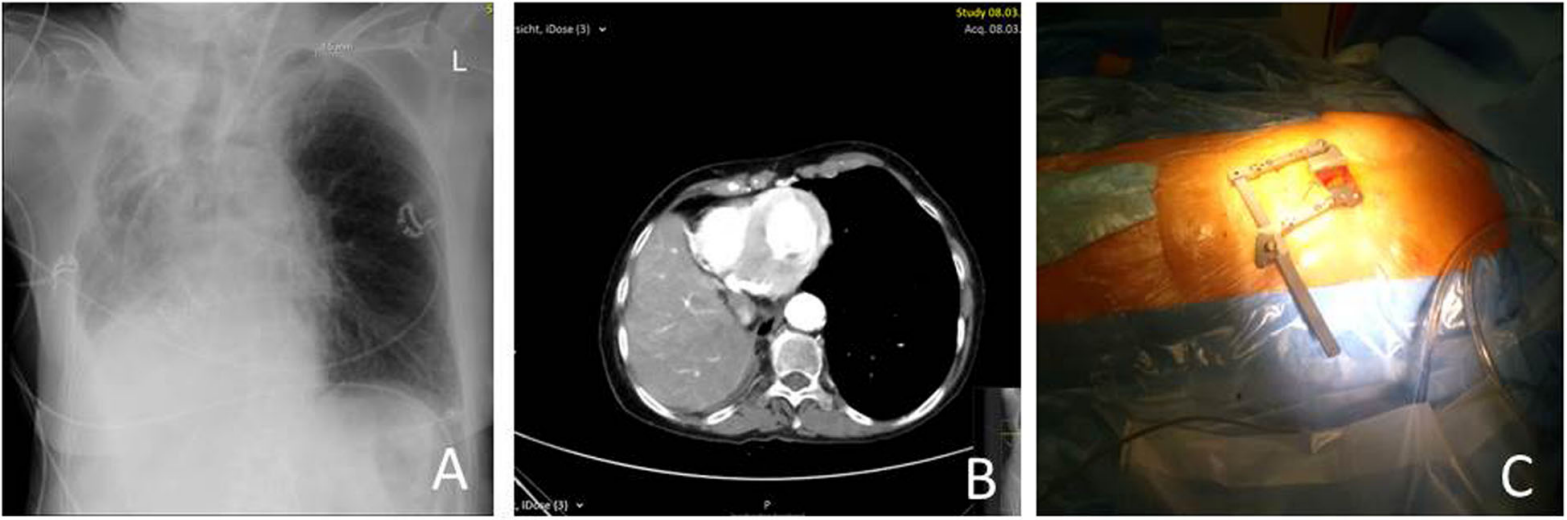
a and b showing rightward shift of the heart. The apex is located below the sternum. c shows the subxiphoidal incision through which TA-TAVR was performed. Arrow on the patient’s chest marks location of the apex as identified by the surgeon by means of transthoracic echocardiography
